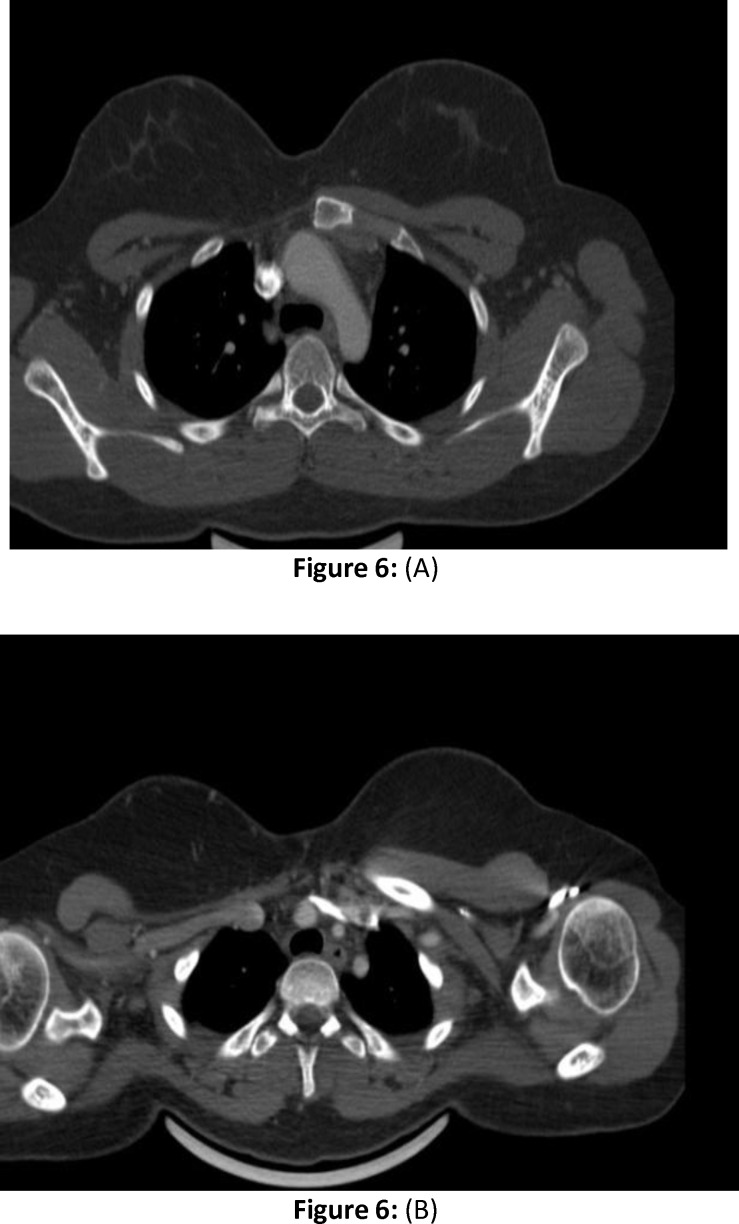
Post operative axial CT scan (A and B) following treatment, which show no mass lesion in operated bed of right clavicular resection. Sternal margin is also smooth
